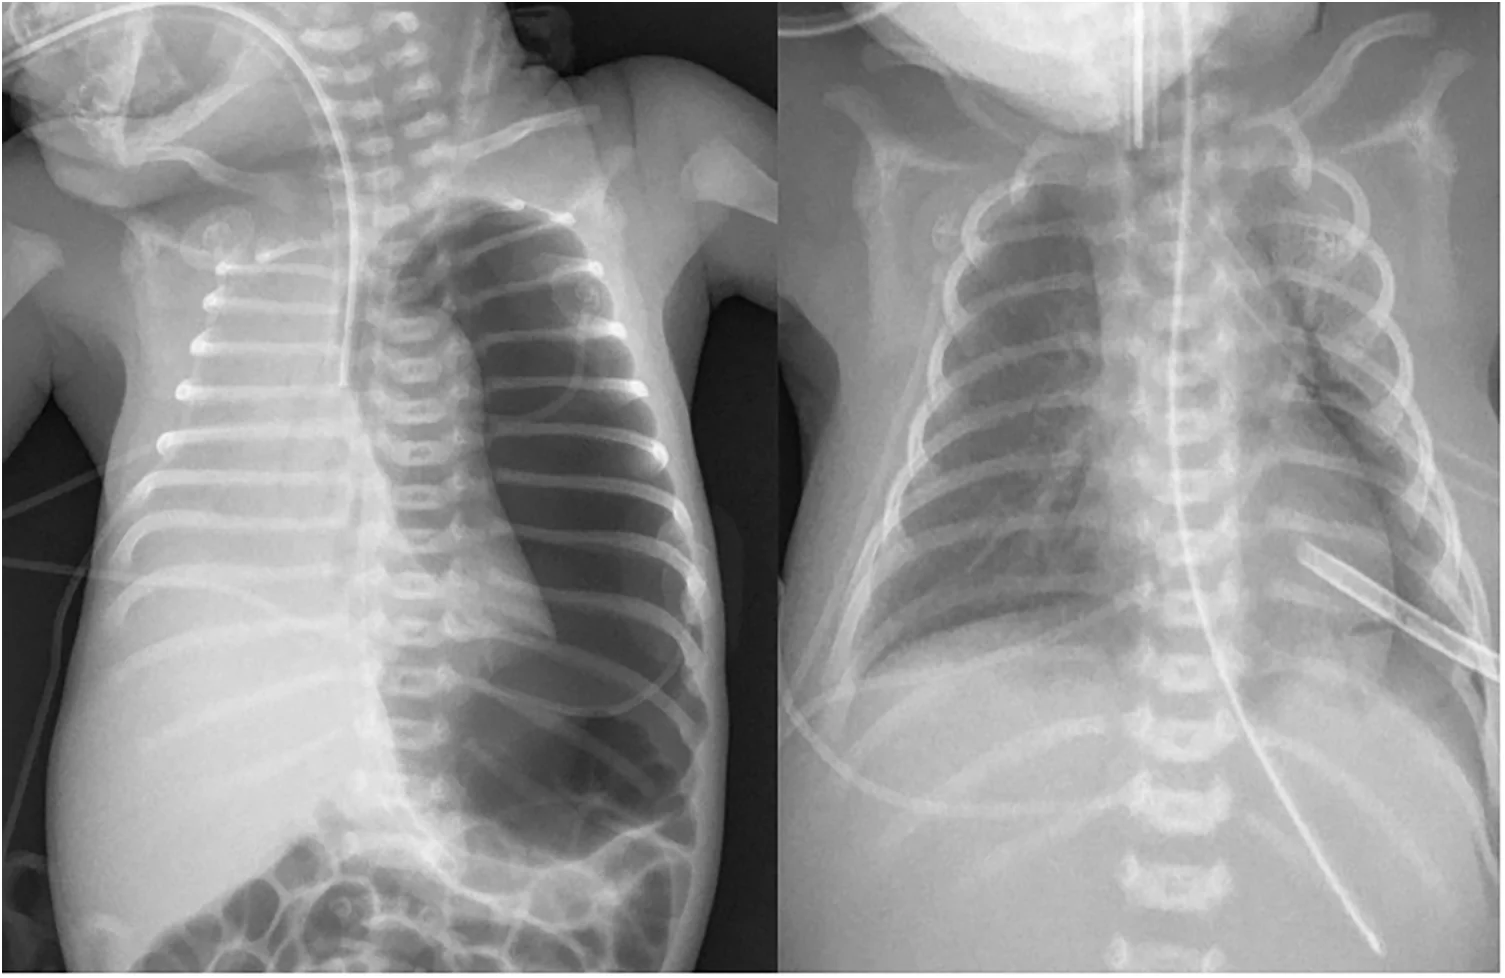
X-ray taken before and after drainage of the pleural cavity.
